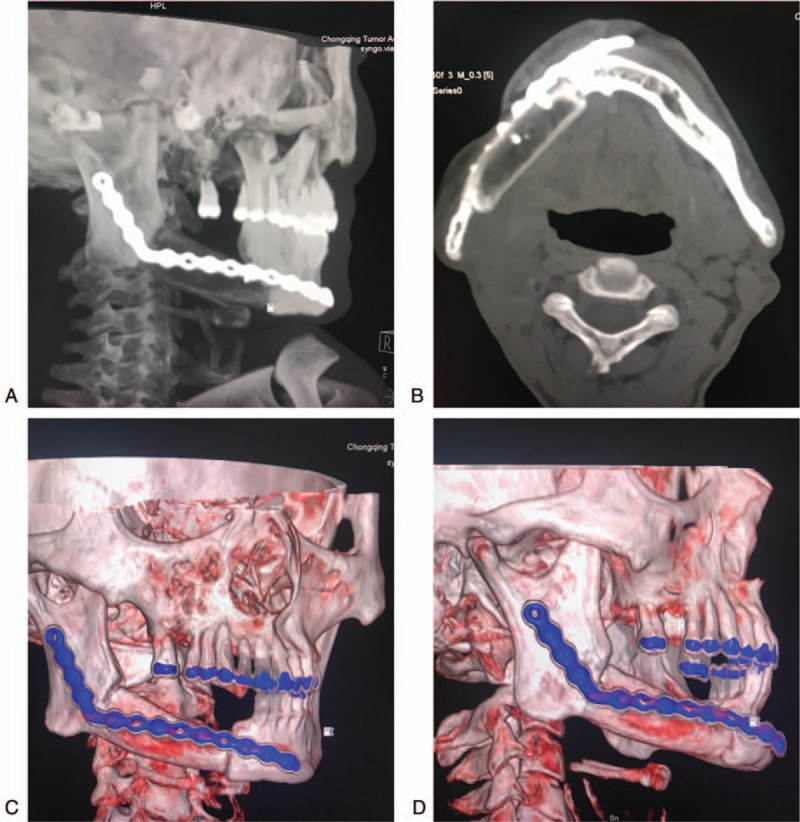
6-month follow-up after surgery: Three-dimensional reconstruction computed tomography of mandible showed the iliac crest bone was connected with the alveolar bone of the mandible, the height of the iliac crest bone was sufficient.
